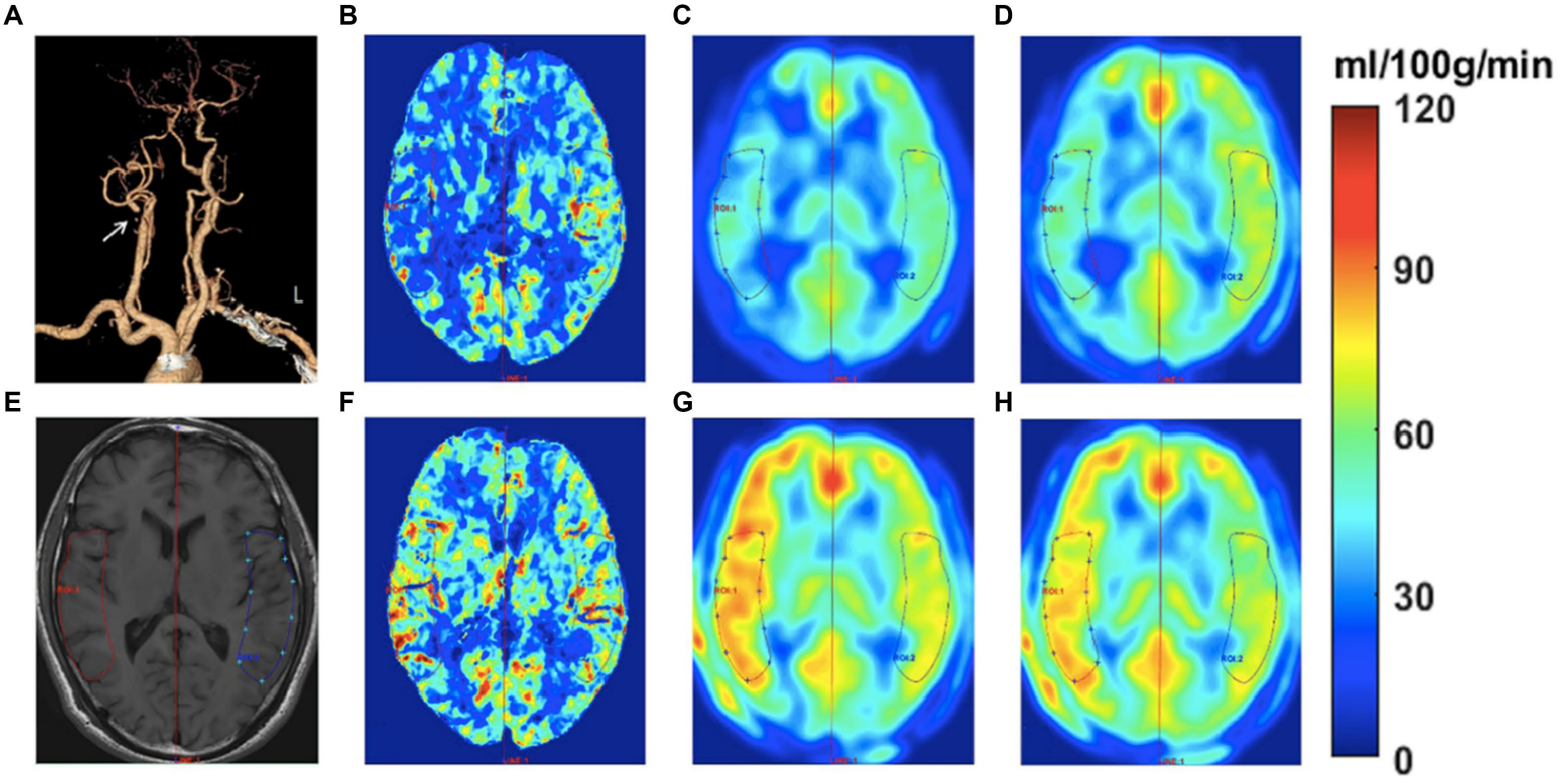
Images of a 58-year-old man with transient ischemic attack (TIA) for half a year. The CTA (A) shows occlusive disease at the right proximal internal carotid artery (see white arrow). The ROIs were drawn on the T1W image to measure the CBF at bilateral sides (E). The CBF color-coded maps of preoperative and postoperative CTP, ASL1.5, and ASL2.0 are shown in B-D and F-H, respectively. The perioperative ASL-CBF maps (C,D,G,H) were visually comparable with the CTP-CBF maps (B,F). The preoperative ASL-CBF maps (C,D) show lower CBF values compared to CTP-CBF (B) on the occlusive side. In contrast, the postoperative ASL-CBF maps (G,H) show higher CBF values than CTP-CBF (F) on the occlusive side.
